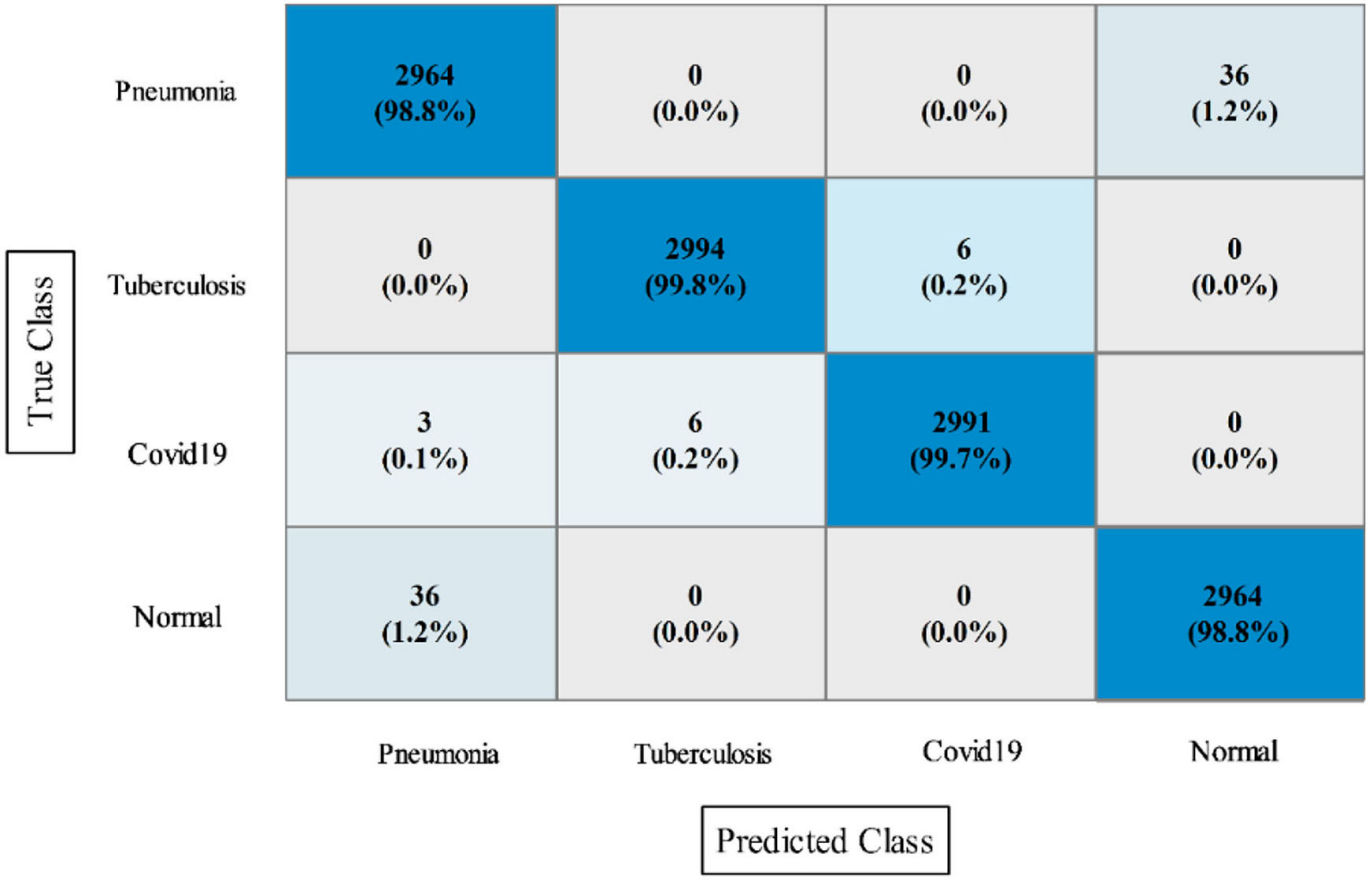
Confusion matrix of WNN for the proposed fusion on the chest X-ray dataset.
